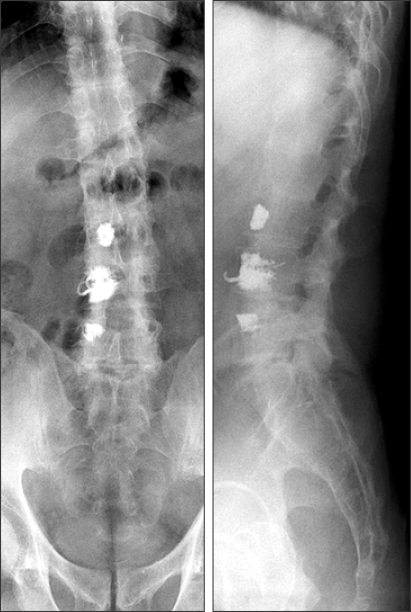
X-ray at final follow-up (2.4 years after second operation) showed interbody fusion at L4-L5 and T10-T11.
